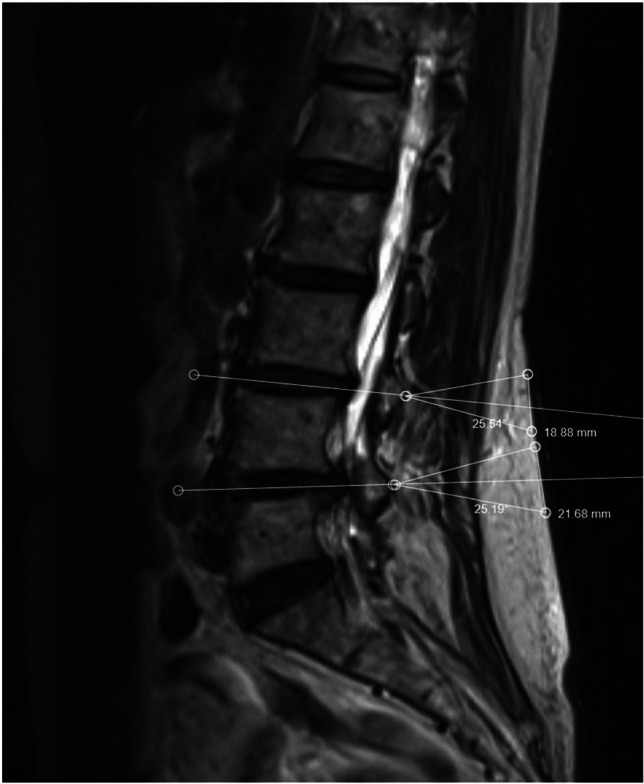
Two level method depicted in preoperative planning based on the T2-weighted sagittal image showing the target level and lamina of interest. Incisions that were within 4–5 mm of each other were typically combined and used for the second level, for a total of 3 incisions
